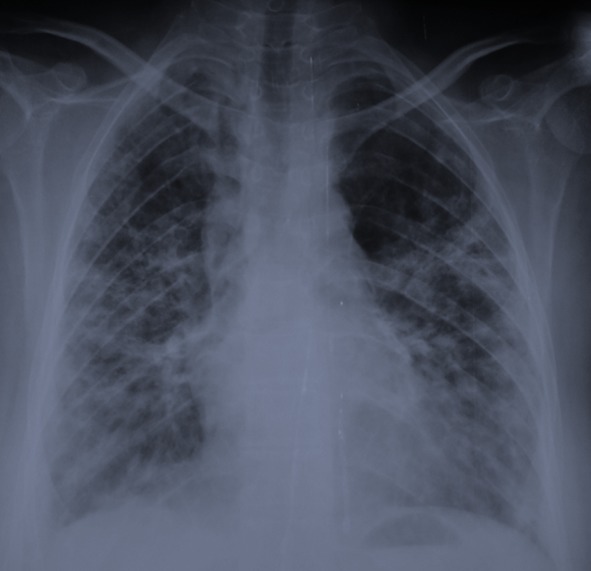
Radiographie thoracique de face: opacités réticulo nodulaires bilatérales en rapport avec un syndrome interstitial
